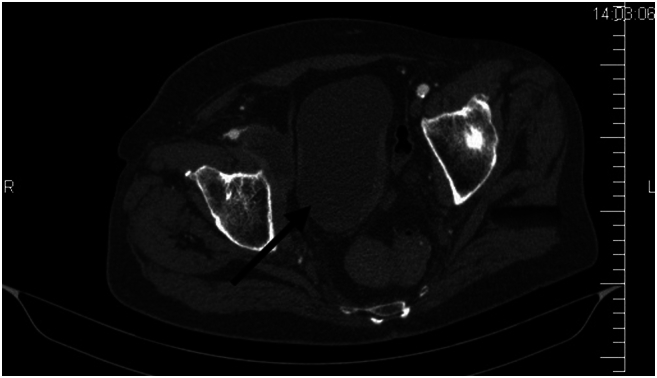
CT scan revealing a neoplastic mass within the pelvic cavity. The arrow points to the mass, which is compressing the right iliac vein.
